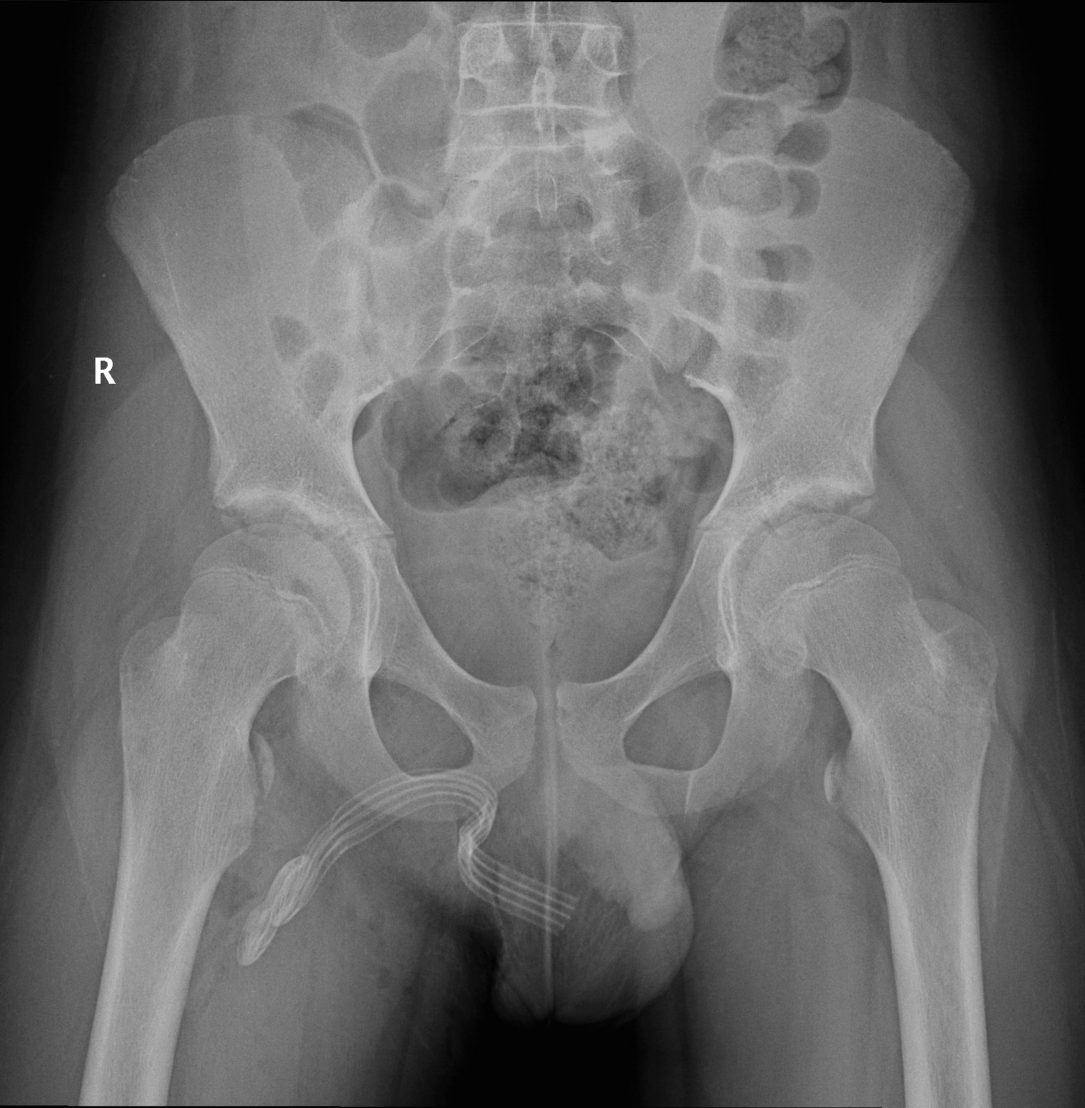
Pelvic X-ray after intraoperative exploration
